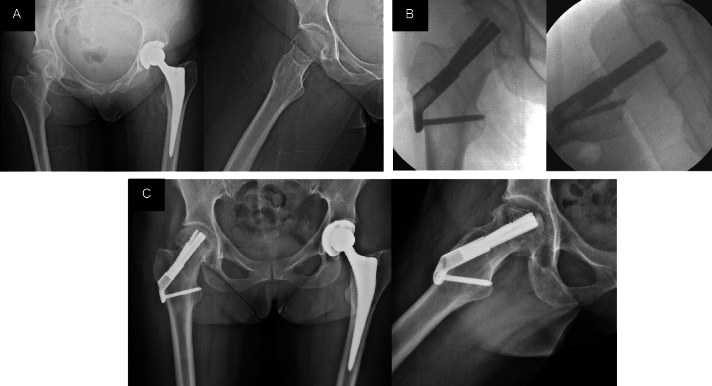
(A) AP and axial hip radiographs taken in the emergency department. (B) Intraoperative fluoroscopy controls with AP and axial views. (C) AP and axial hip radiographs at the 6-month follow-up. Articular surface collapse and protrusion of the bolt and anti-rotational screw are observed.
